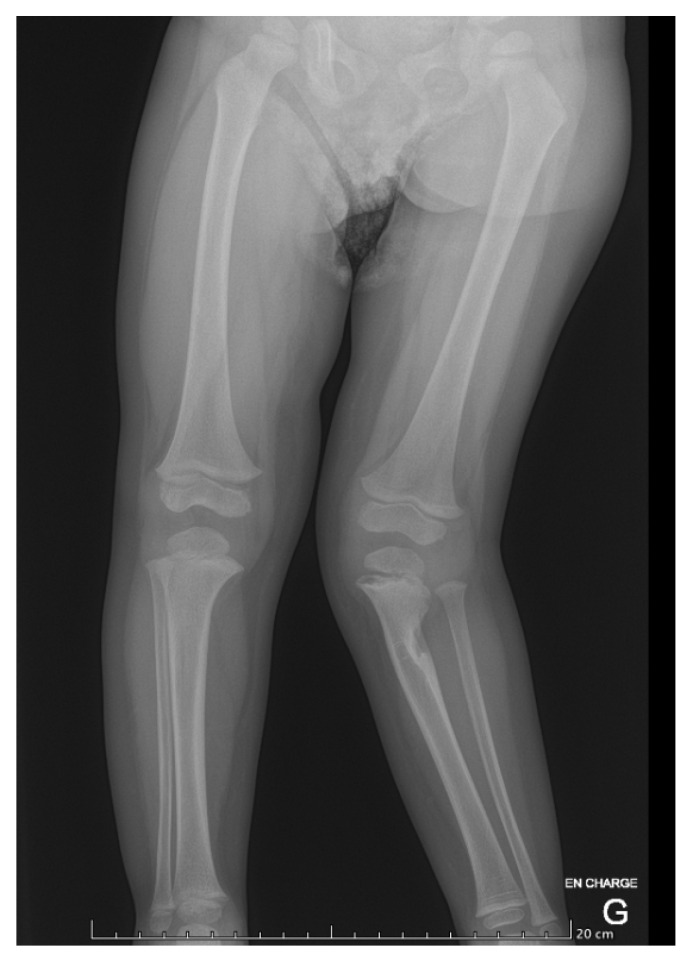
Radiographic imaging (anteroposterior weight-bearing view) demonstrating an unusual valgus deformity of the left proximal tibia in FFCD, characterised by a lateral radiolucent metaphyseal defect with surrounding cortical condensation and irregularity. The letter “G” on the radiograph indicates the left side (“gauche” in French).
